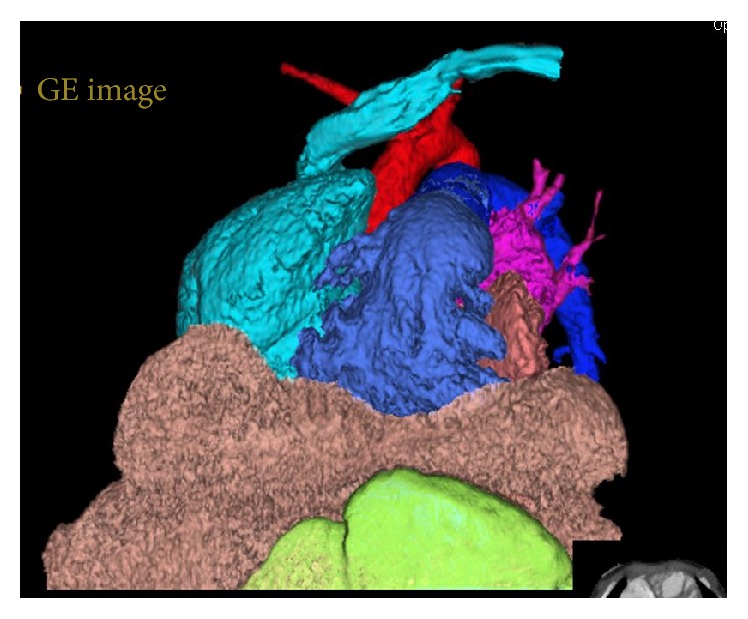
3D color coded cardiac CTA frontal projection shows bilateral focal liver (tan) herniating up through the anteromedial aspect of the diaphragm in front of the right atrium (purple) and right atrium (light blue).
